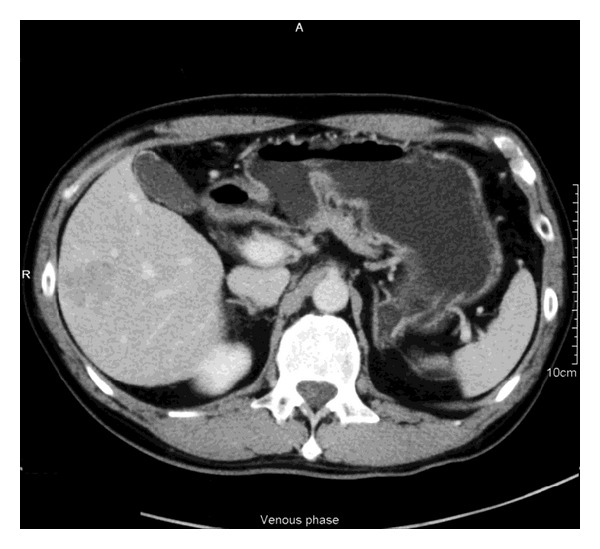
Preoperative CT scan showing the hepatocellular carcinoma at segment 6. The stomach was unremarkable. No gas was noted in the portal vein.
